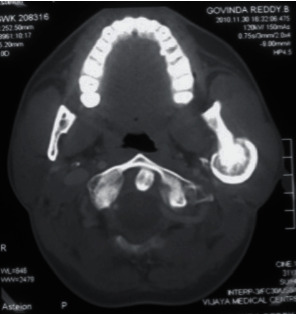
Computed tomography axial section showed a well-defined osseous bony outgrowth arising from the posterior edge of the left ramus of the mandible. The lesion showed central medullary with sclerosed cortical bone at the periphery, continuing with the condyle leading to mild elongation and giving a mushroom-shaped appearance on the axial section.
